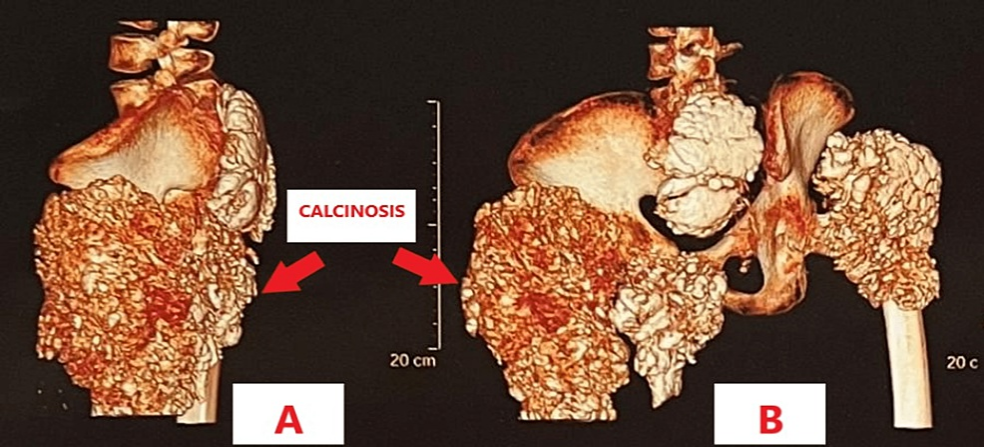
3D CT scan of pelvis with both hips showing calcinosis. 3A: Lateral View 3B: Posteroanterior (PA) View
